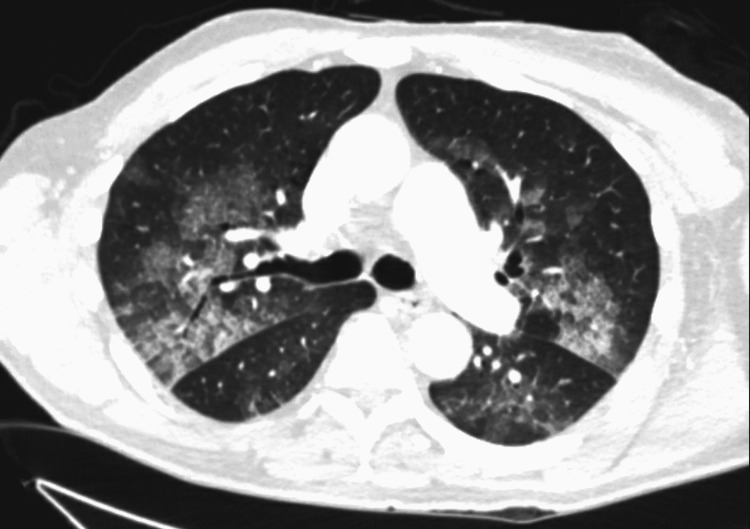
Ground glass opacities in Chest CT
